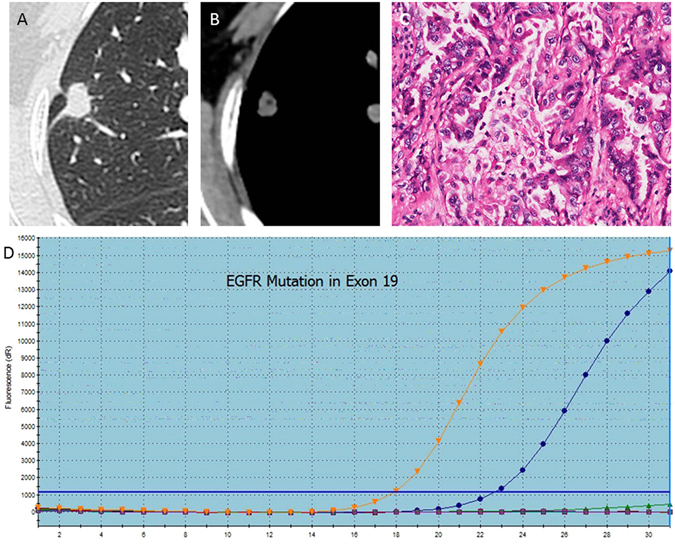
A 62-year-old woman with EGFR mutation of lung adenocarcinoma in the right upper lobe, where the pathological type (A) is EGFR mutation in exon 19, and CT images (B,C) show a small maximum-diameter lump about 14 mm with obvious pleural retraction and absence of fibrosis. The PCR picture (D) shows that the blue line is similar to the upper line, indicating that the subtype of mutation is exon 19 delection.
